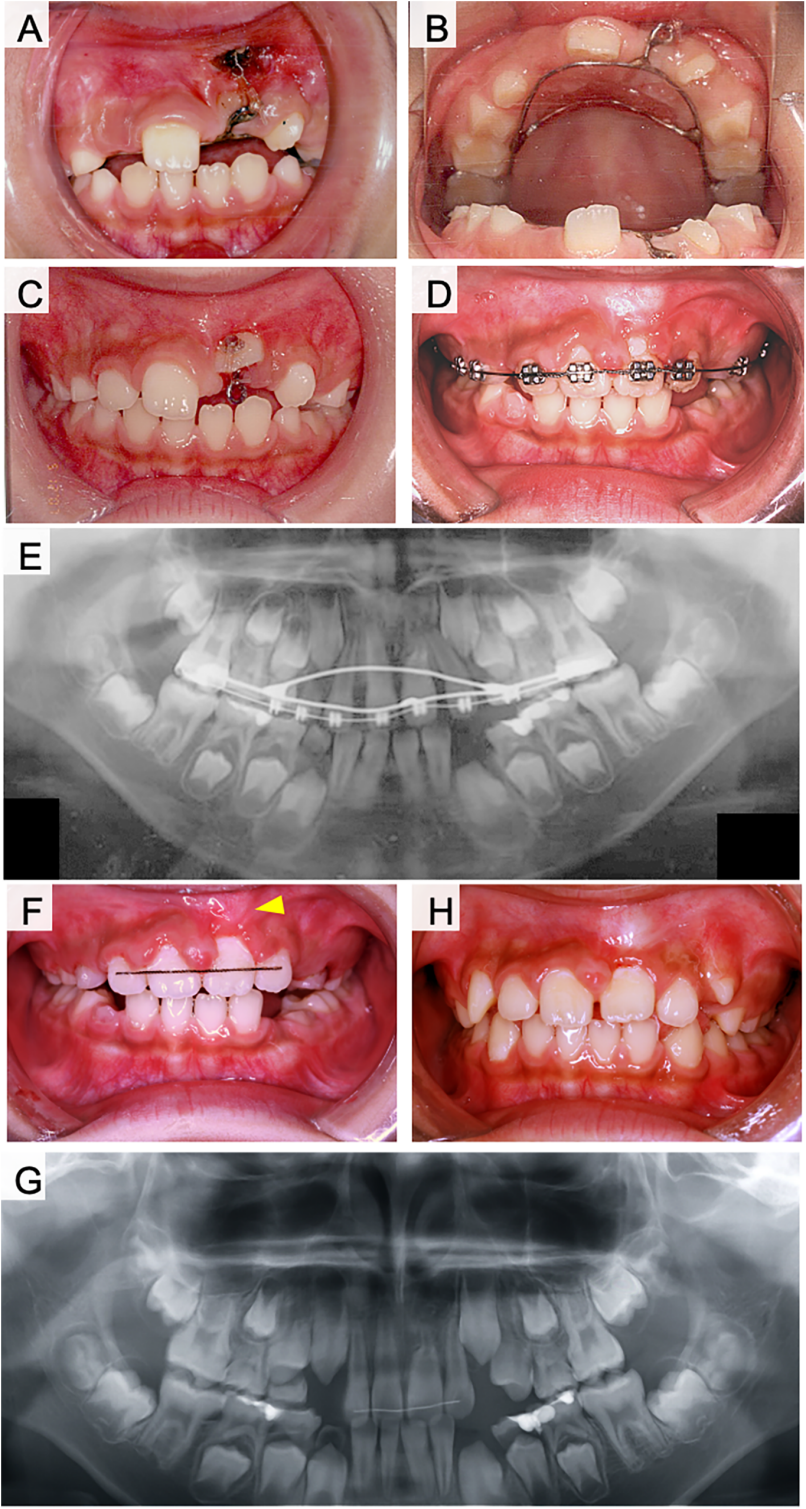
Treatment progress with fenestration and traction of the impacted tooth in case 2. (A) Frontal view of intraoral photograph at the start of the fenestration and traction. (B) Maxillary occlusal view of intraoral photograph at the start of the fenestration and traction. (C) Six months after the start of traction. (D) One year and five months after the start of traction. (E) Panoramic radiograph after one year and five months of traction. (F) During the retention period. (G) Panoramic radiograph of post-treatment. (H) One year and six months after the end of treatment.
